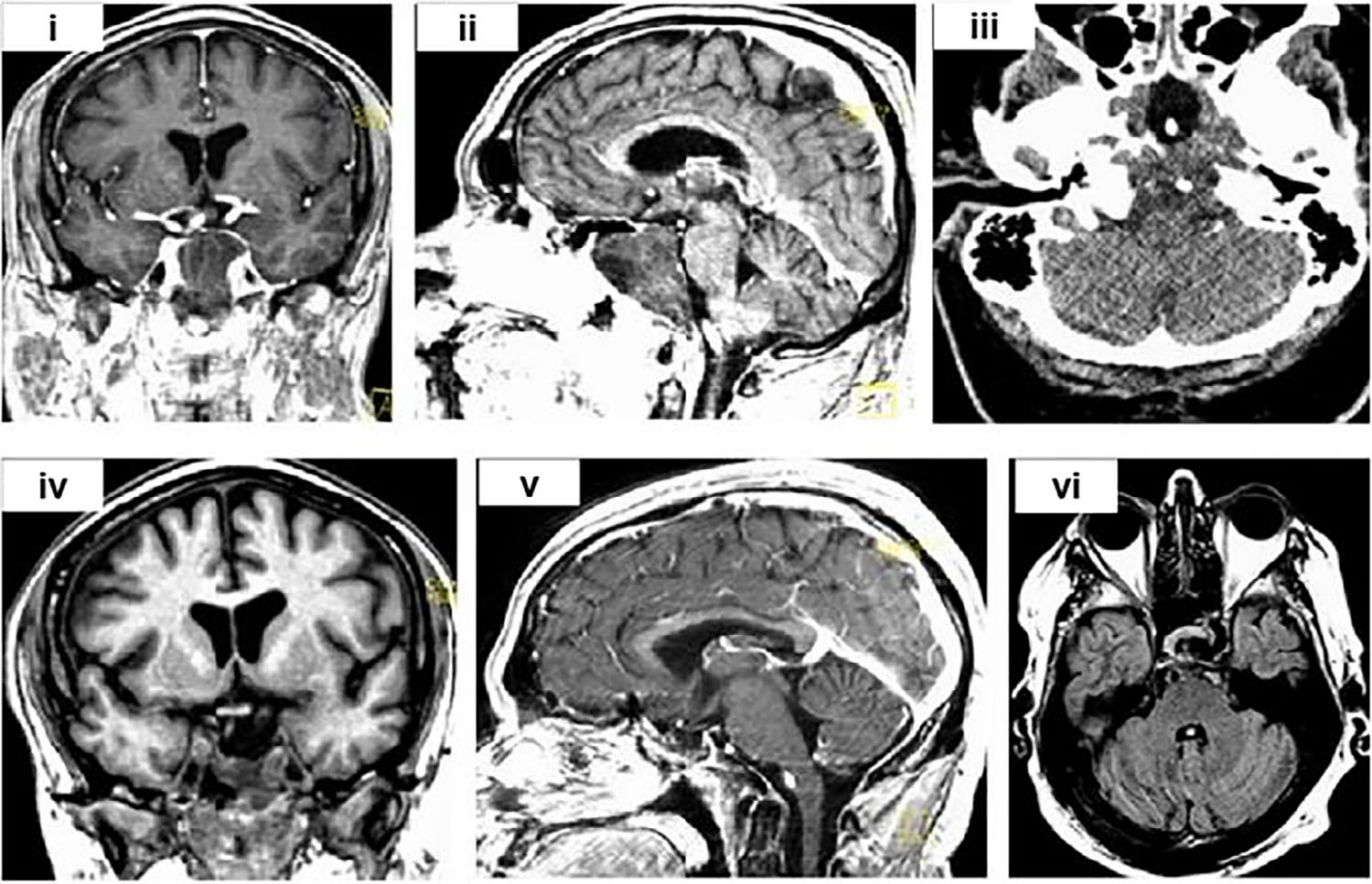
Cryosurgery for brain tumors. MRI images of a large pituitary tumor (i,ii). CT image with the formation of ice balls (shown as black voids) around the probe in the tumor (iii). Coronal, sagittal, and axial views of the MRI, with a significant reduction in tumor size (iv,vi). Reproduced under the terms of the CC‐BY 4.0 license.^[^
